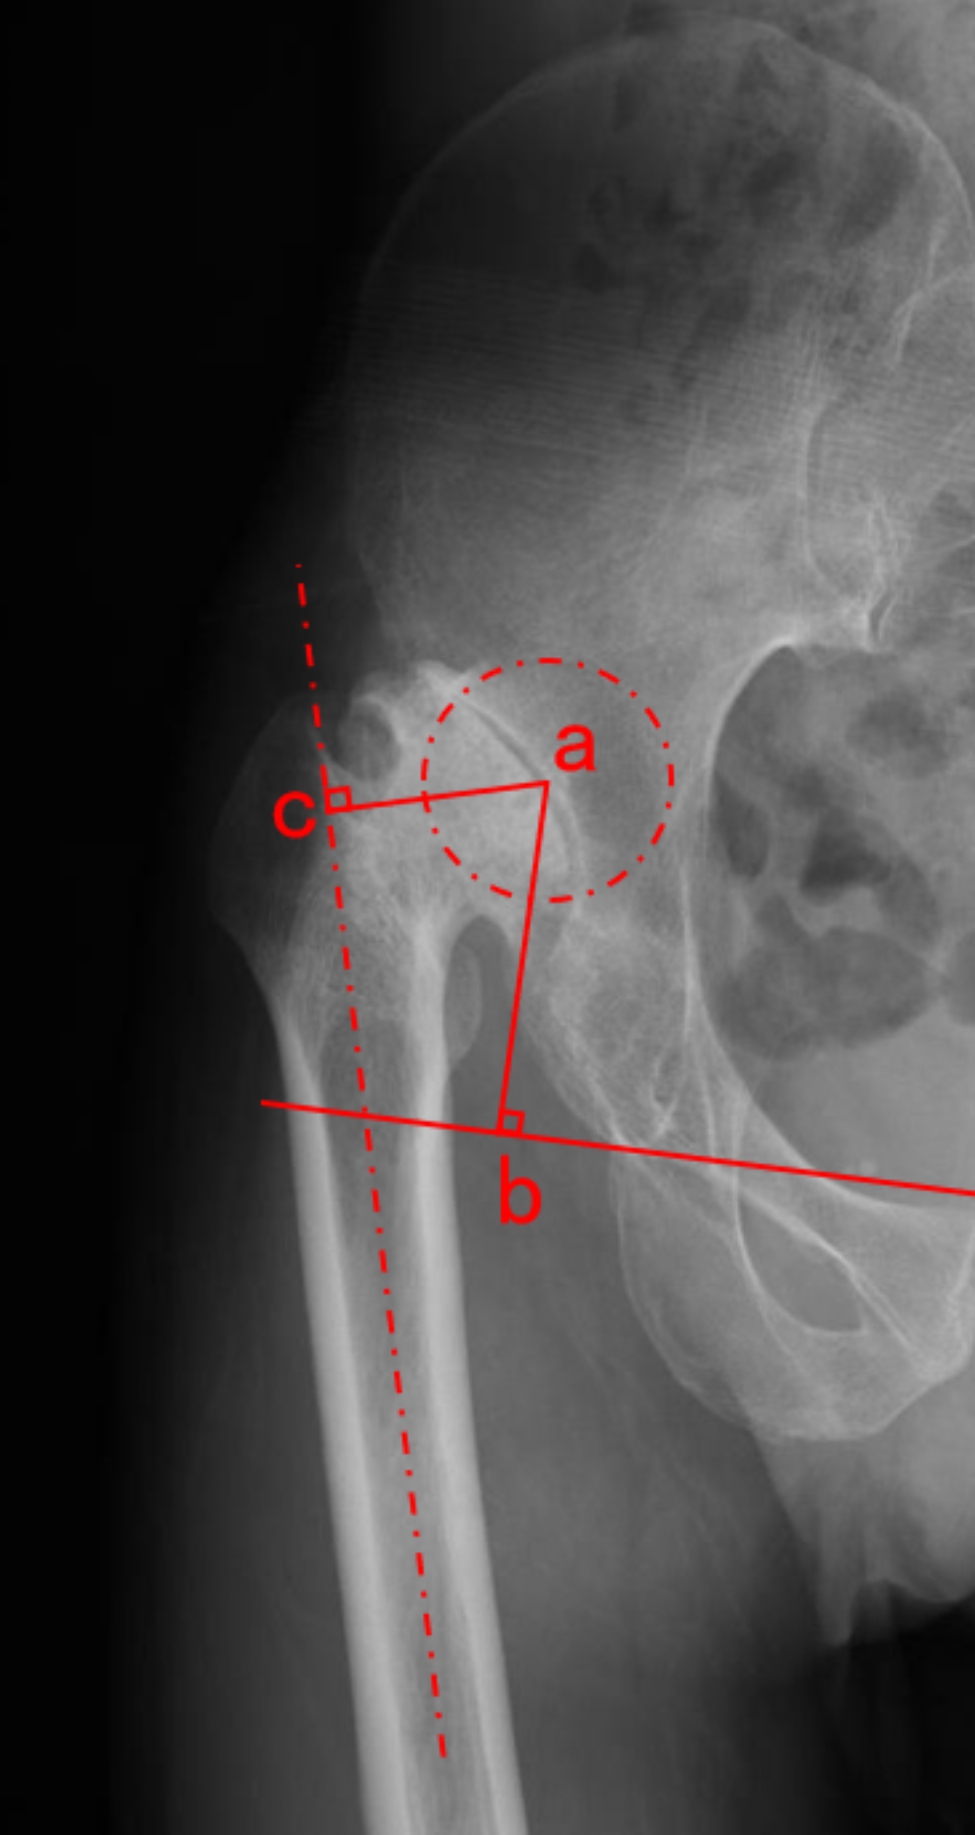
The center of rotation (COR) height and femoral offset (FO) were measured on the AP pelvic radiograph. The COR height (ab): the perpendicular distance between the center of rotation and the interteardrop line. FO (ac): the perpendicular distance between the center of rotation and the axis of the femoral shaft
